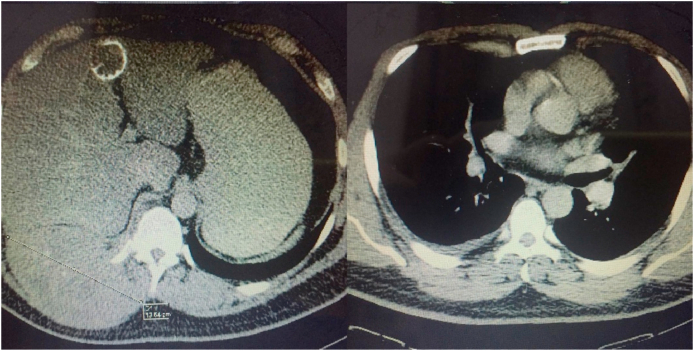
Whole CT-scan performed on 30^th^ December 2019 showing a calcified cystic structure.
